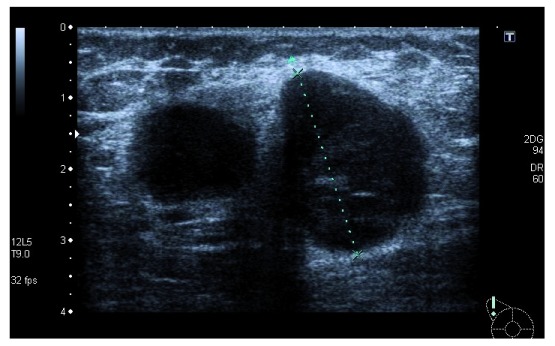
Ultrasound second look and fine-needle aspirate biopsy of the second lesion.
